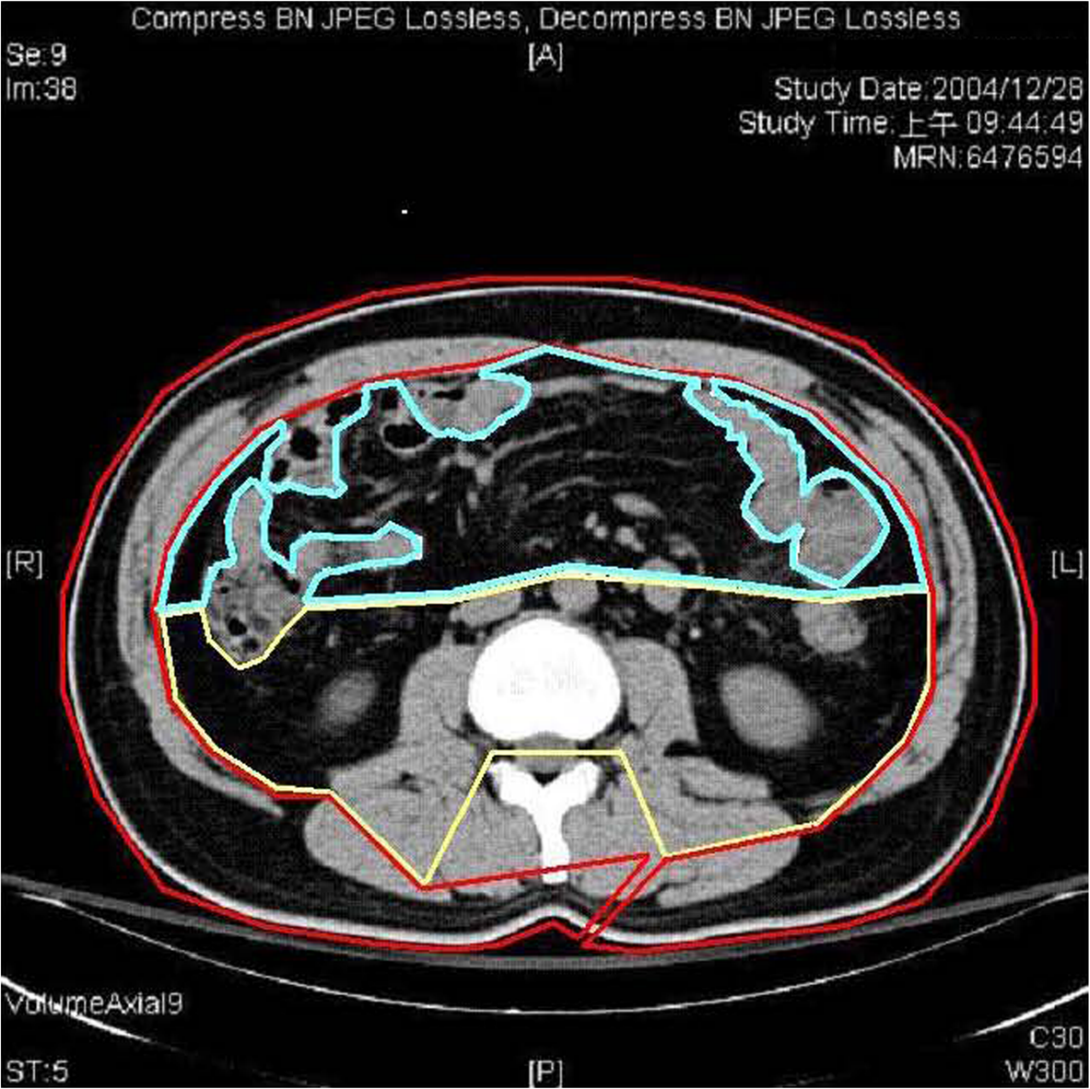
The CT of abdomen shows measurement of intra-peritoneal fat (blue line), retro-peritoneal fat (yellow line) and subcutaneous fat (red line).
